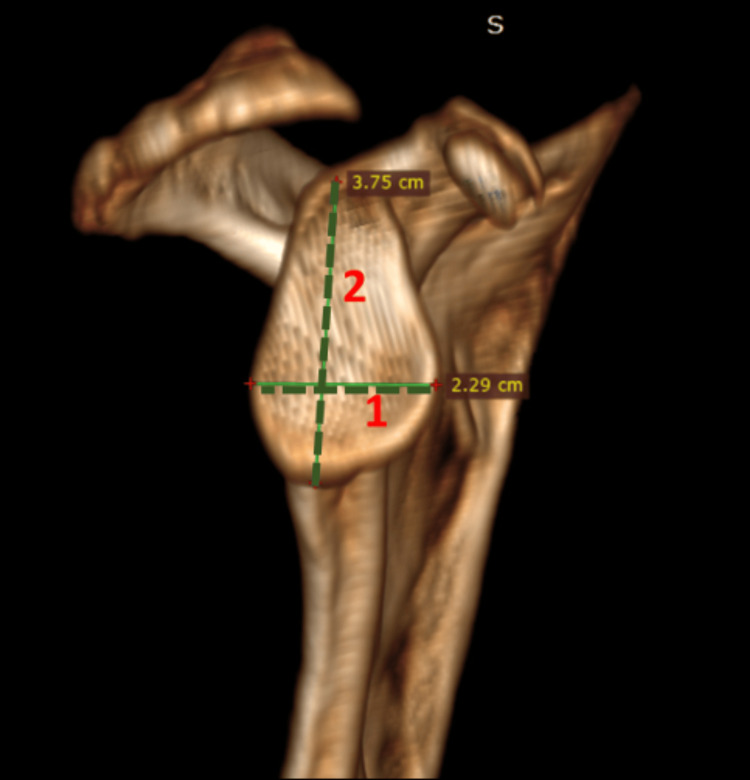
3D CT lateral view of the right scapula showing measurement of the glenoid fossa 1: maximum anteroposterior diameter of the glenoid (glenoid width); 2: maximum supero-inferior diameter (glenoid height) CT: computed tomography
